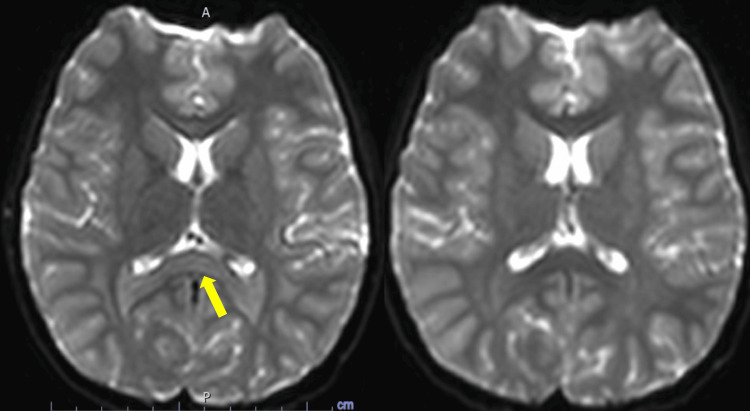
Case 2: Axial DWI of the brain (A) Initial MRI (September 2019): diffusion-restricted hyperintensity involving the splenium of the corpus callosum. (B) Follow-up MRI (October 2019): complete resolution of the diffusion restriction in the splenium of the corpus callosum. DWI, diffusion-weighted imaging
